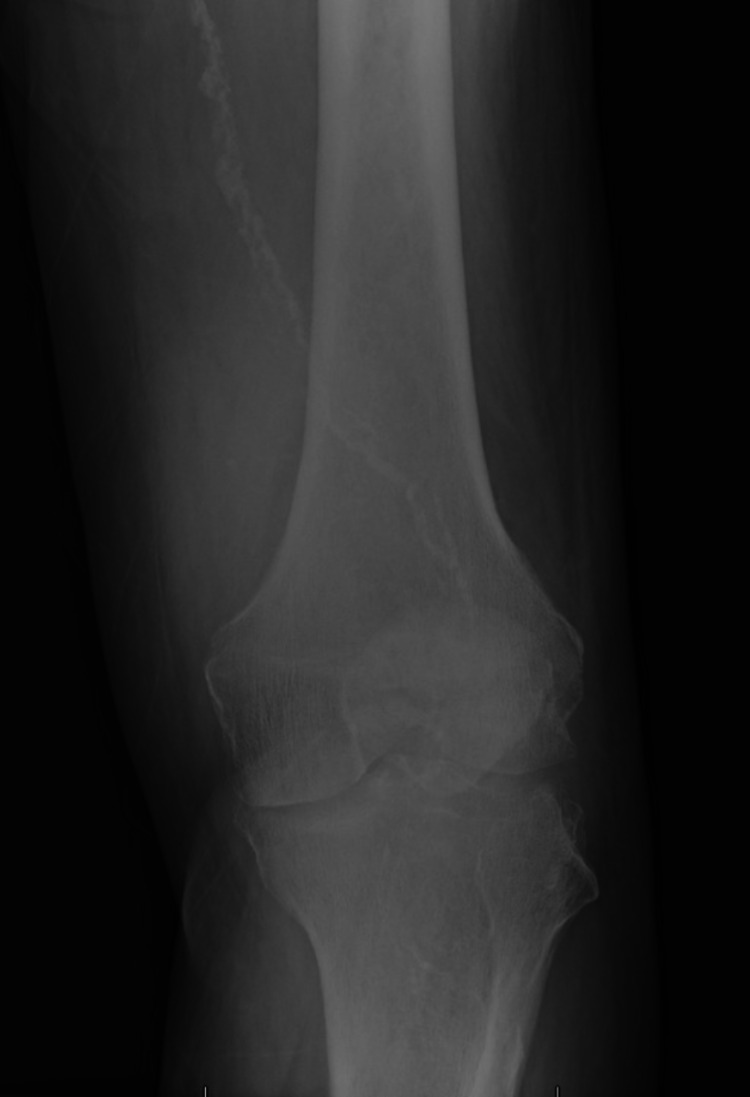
X-ray left knee (A/P view)
